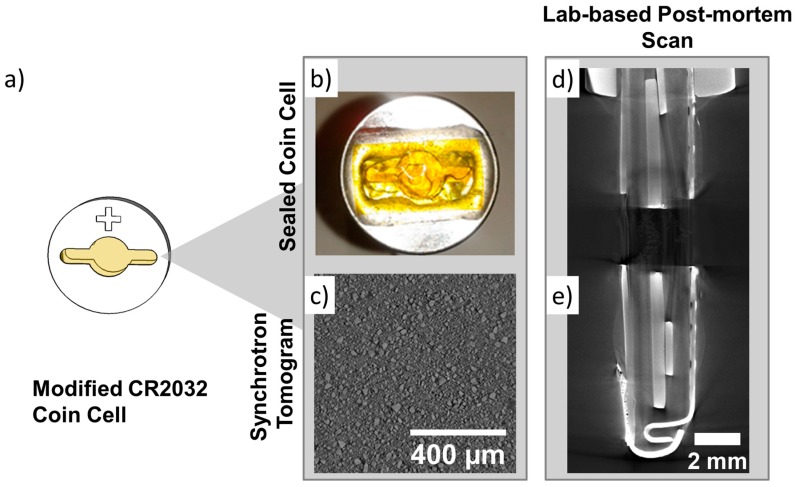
(a) Modified CR2032 coin cell rendering and (b) image of the Kapton window attached to the cell. (c) Reconstructed slice of a LiMnO_2_ electrode acquired at a synchrotron facility and (d,e) post-mortem CT scan of the entire cell showing its components.
